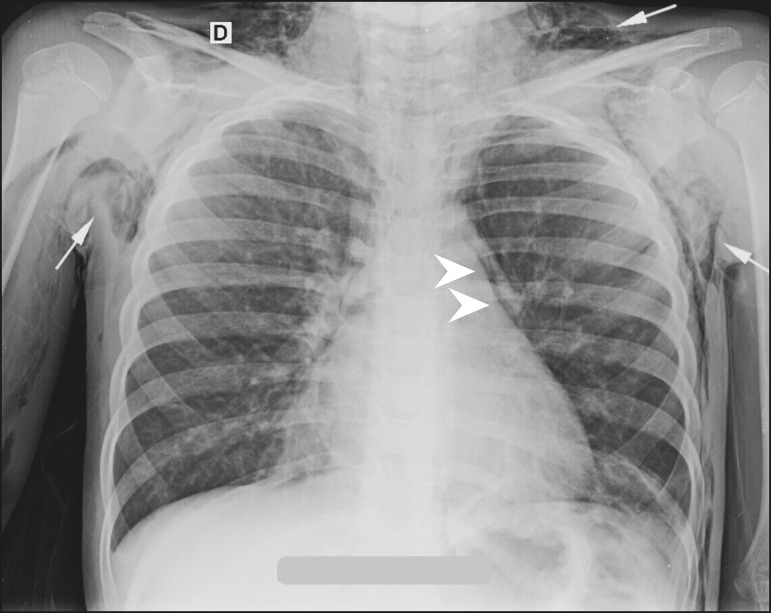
Posteroanterior chest X-ray showing pneumomediastinum (arrowheads), together
